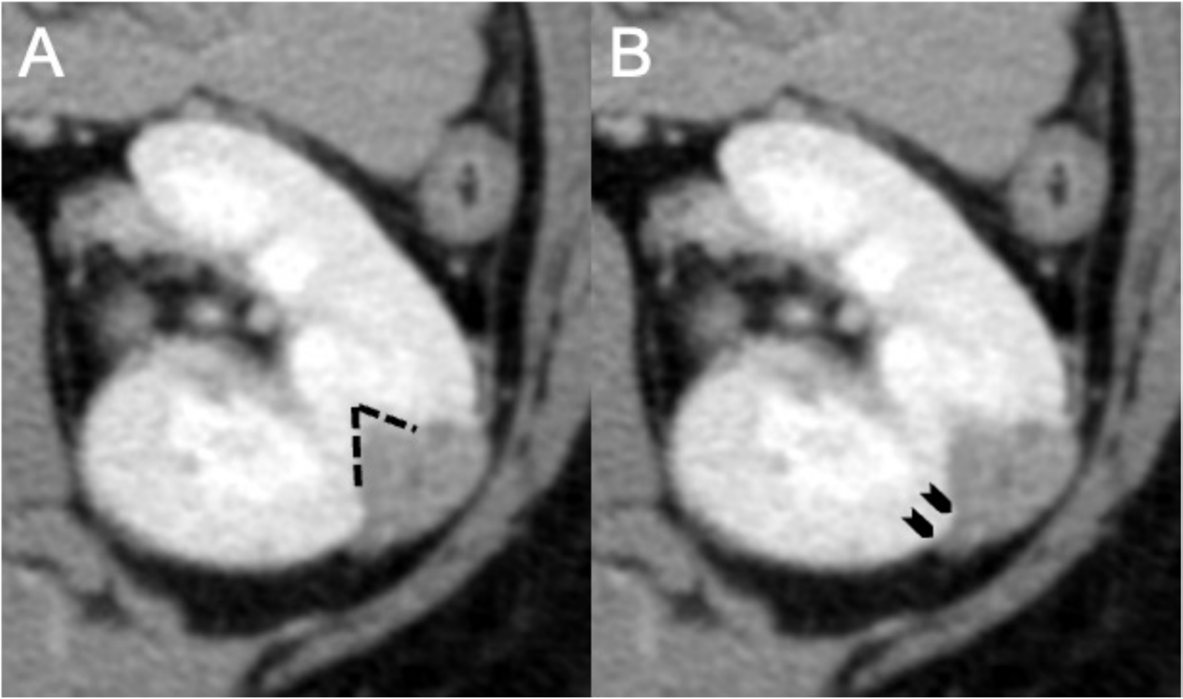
Representative images showing the appearance of AI and OBS on CECT. A The AI in the parenchymal phase was defined as an angle of 90° or less (black lines). (B) A contact length ≥ 3 mm of the bulging portion of the renal tumor onto the renal surface was indicative of a positive OBS (black arrows). AI, angular interface; OBS, overflowing beer sign; CECT, contrast-enhanced computed tomography
